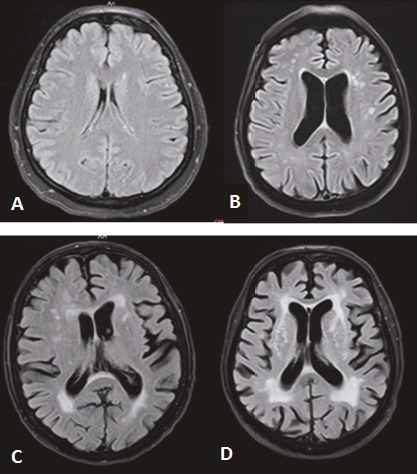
Fazekas classification system was demonstrated by MR in FLAIR sequence. A) Fazekas 0: normal white matter intensity, none or a single punctate lesion. B) Fazekas 1: multiple punctate lesions. C) Fazekas 2: beginning of confluency of lesions. D) Fazekas 3: large confluent lesions.
